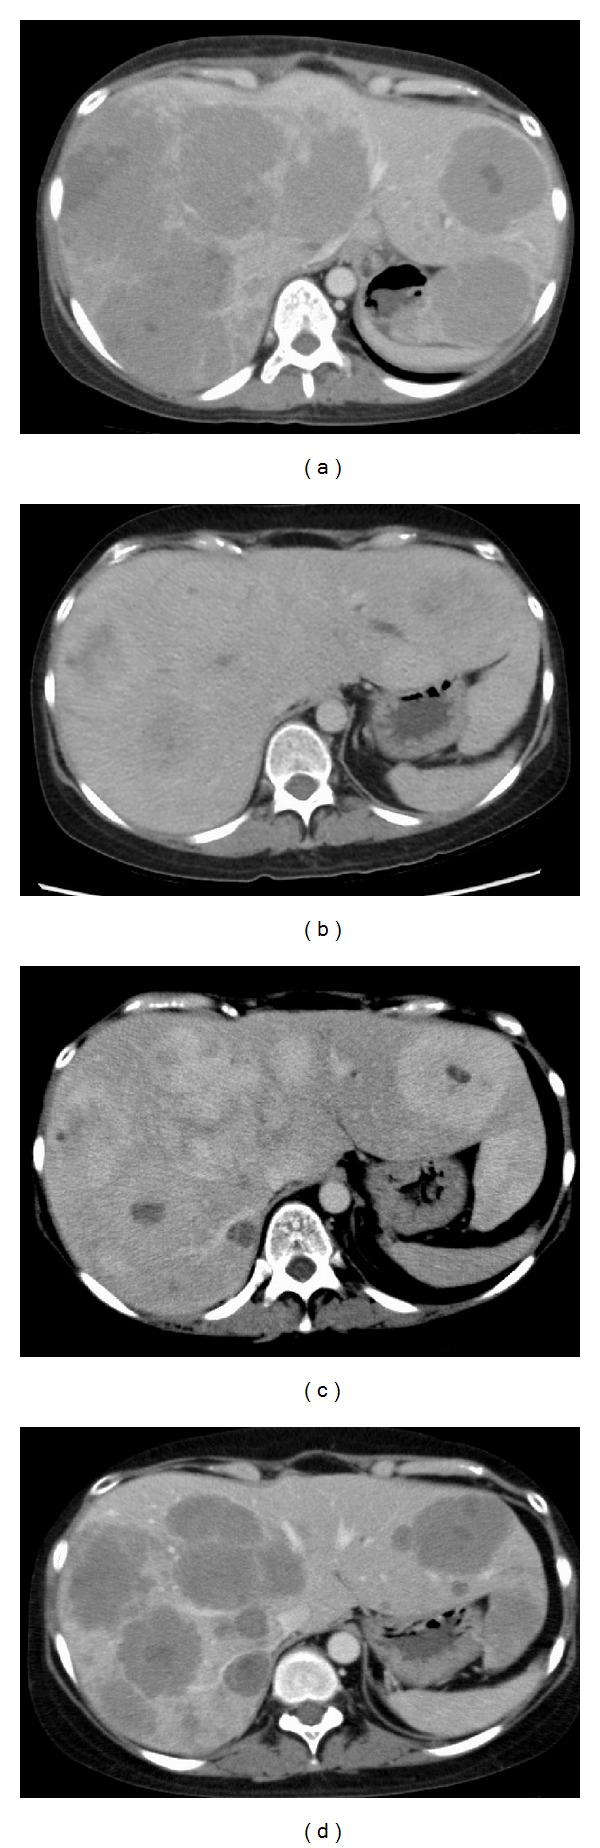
(a) Computed tomography (CT) before first temsirolimus administration. There are multiple metastatic tumors in liver. All tumors are enhanced weakly (64 Hounsfield units). (b) CT 2 months after temsirolimus administration. Most tumors shrink. (c) CT 5 months after temsirolimus administration. Metastatic tumors grow again. All tumors are enhanced strongly (137 Hounsfield units). (d) CT 3 weeks after sunitinib readministration. All tumors turned to be hypovascular again (67 Hounsfield units).
